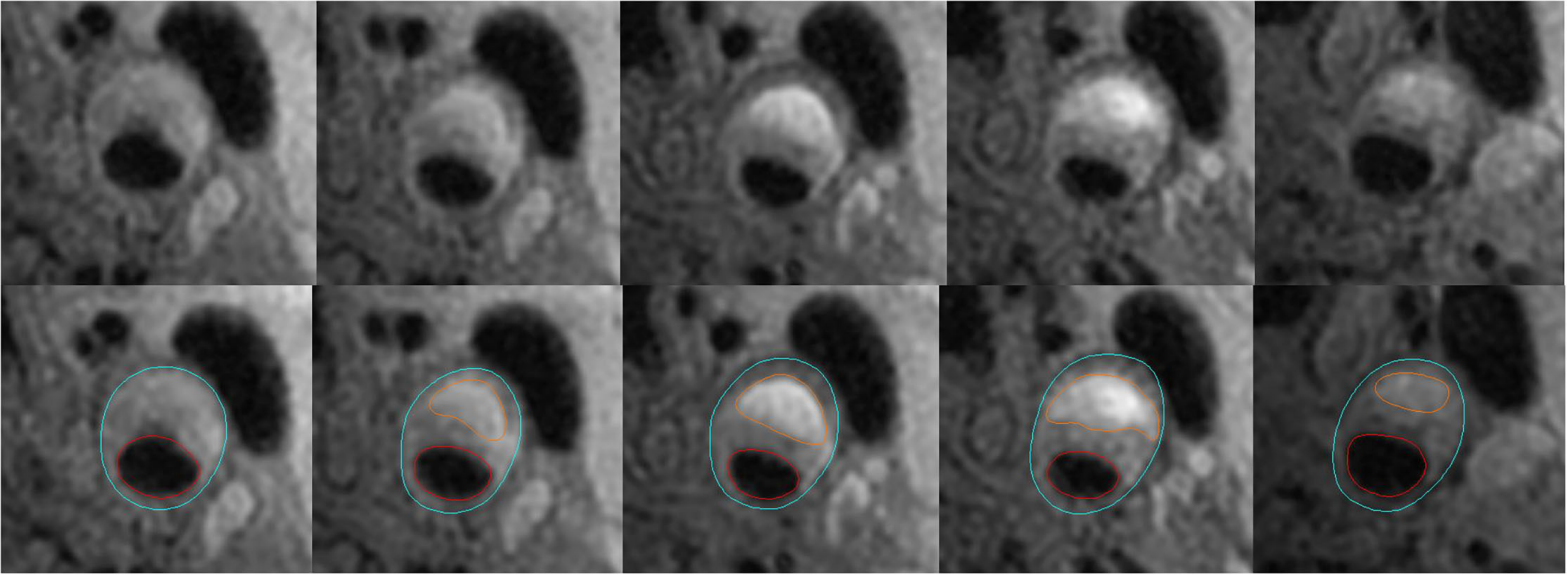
Example for measurements of carotid plaque-containing vessel wall volume and IPH volume. The figure shows that the lumen in red and outer wall boundary in blue of the carotid artery are identified and outlined on T1-weighted images. Carotid plaque-containing vessel wall volume is calculated by multiplying the slice thickness (2 mm) by the sum of the areas between the red and blue circles in slices with a vessel wall thickness of at least 2 mm. IPH volume is calculated by multiplying the slice thickness (2 mm) by the sum of the areas circled in orange color.
